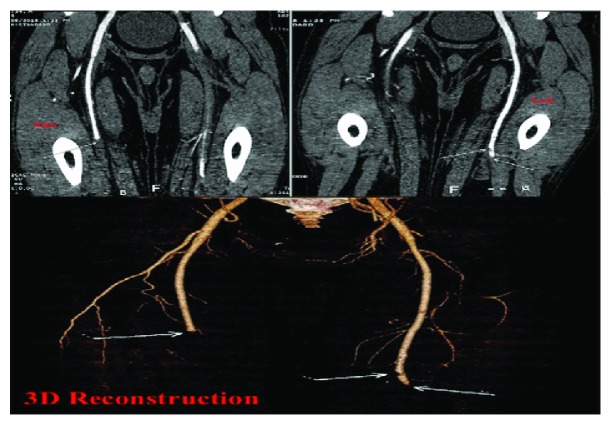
A computed tomography angiogram of bilateral lower extremity: severe occlusive bilateral superficial femoral arteries at the level of the mid thighs and occlusion of the right internal iliac artery. Mild reconstitution is seen on the right side anterior tibial artery with no flow in it seen distal to the ankle. No reconstitution is seen on the left side. No radiographic evidence of collateralization.
